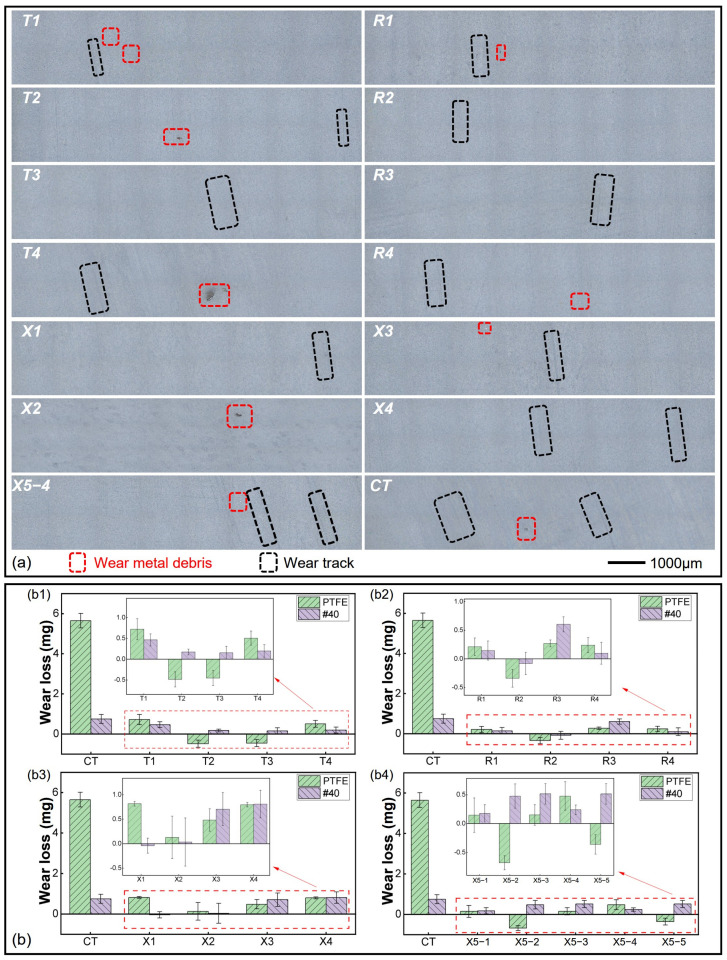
Representative worn surfaces of the PTFE rings and wear losses of different groups after wear tests. (a) Worn surfaces of the PTFE rings after ultrasonic cleaning. (b) Mass losses of the PTFE rings and 40# steel discs: (b1) mass losses of T1–T4; (b2) mass losses of R1–R4; (b3) mass losses of X1–X4; (b4) mass losses of X5-1–X5-5.
